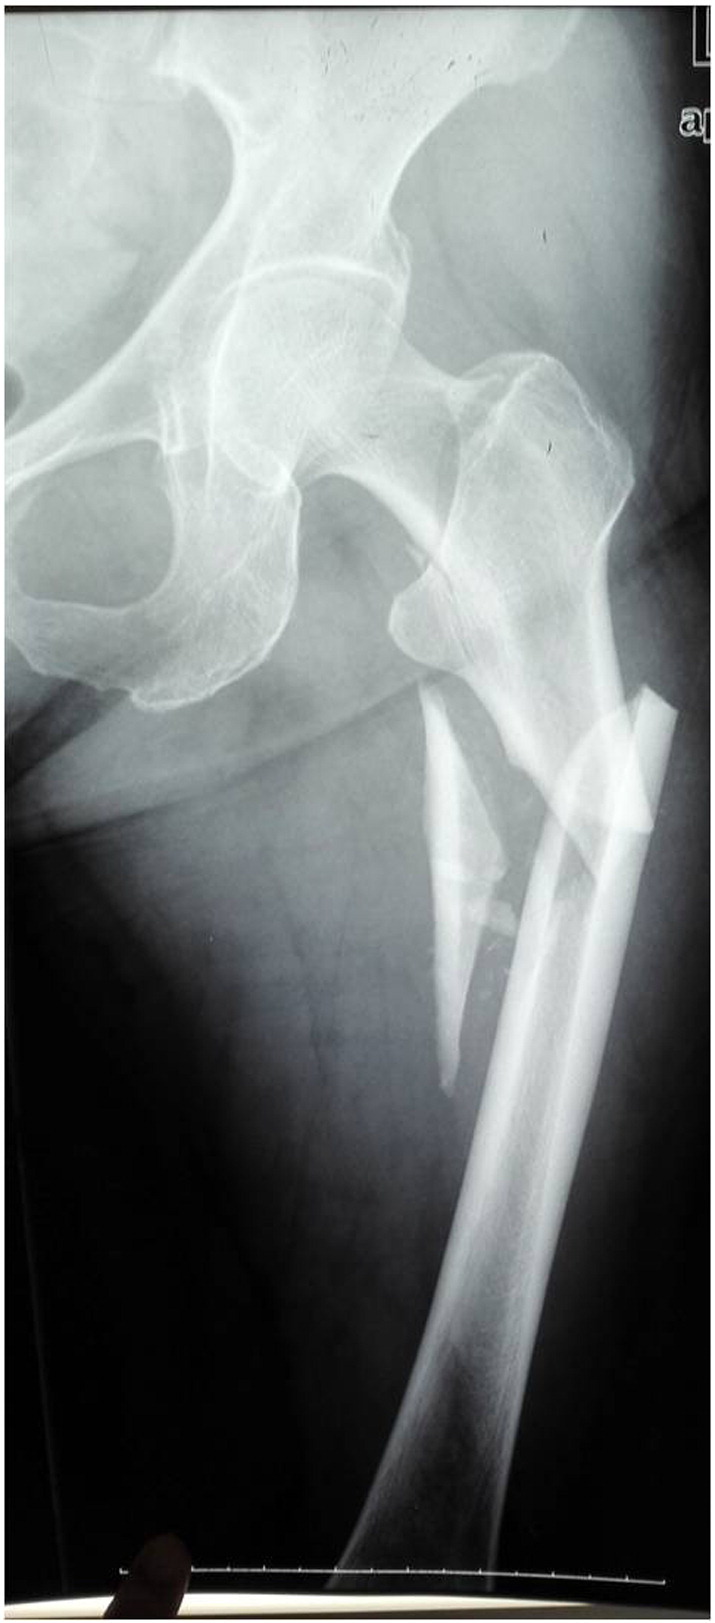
AP radiographic reveal a ipsilateral left femoral neck and sub-trochanteric fracture.
